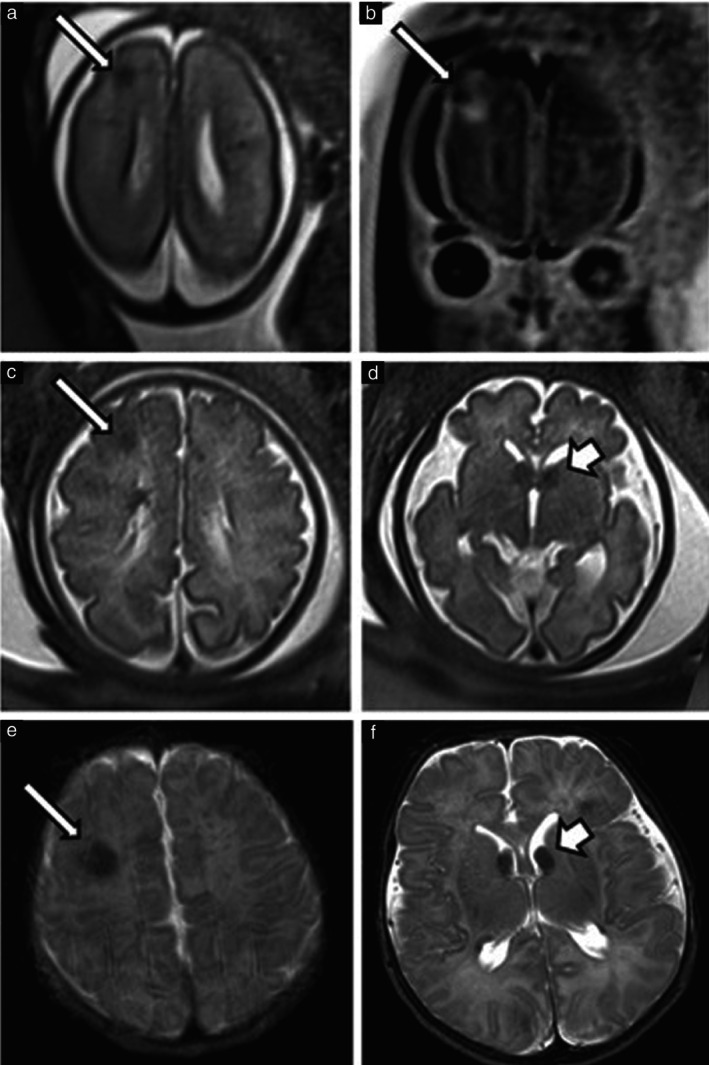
Magnetic resonance imaging (MRI) for assessment of brain lesion severity in single case of tuberous sclerosis complex treated with prenatal sirolimus for > 7 days (initiated at 31 + 4 weeks' gestation). (a,b) Prenatal MRI at 23 weeks showing: (a) axial T2‐weighted image and (b) coronal T2‐weighted image; arrow indicates subcortical tuber. (c,d) Prenatal MRI at 35 weeks showing two axial T2‐weighted images, demonstrating: (c) same subcortical tuber (arrow) as in part (a) and (d) subependymal giant cell astrocytoma (SEGA) (short arrow). (e,f) Postnatal MRI on day 2 after delivery showing two axial T2‐weighted images, demonstrating: (e) the subcortical tuber (arrow) and (f) the SEGA (short arrow), corresponding to the lesions seen prenatally.
